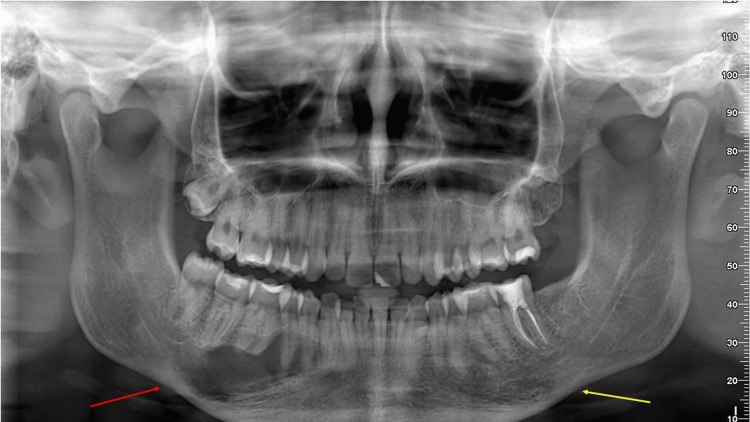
Postoperative radiograph, displaying a significant reduction in the size of the radiolucent lesion in the left body of the mandible (yellow arrow), indicating satisfactory bone healing and ongoing regeneration. In contrast, the radiolucent lesion in the right body of the mandible (red arrow) remains untreated, as surgical intervention was deferred at the patient’s request.
